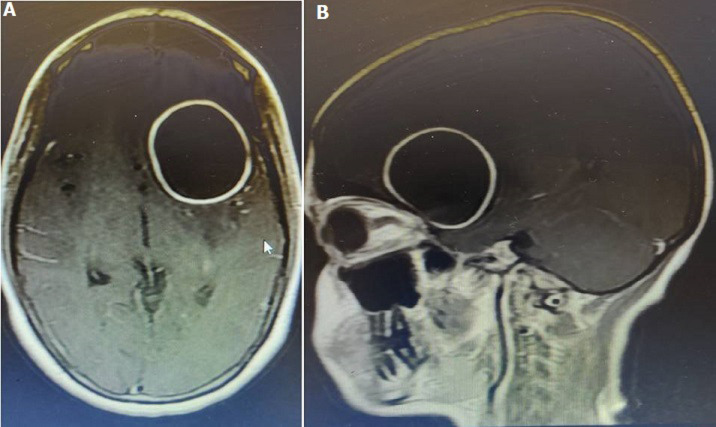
axial view of pre-operative MRI showing well defined circumscribed lesion (A); sagittal view of pre-operative MRI showing well-defined circumscribed lesion (B)
